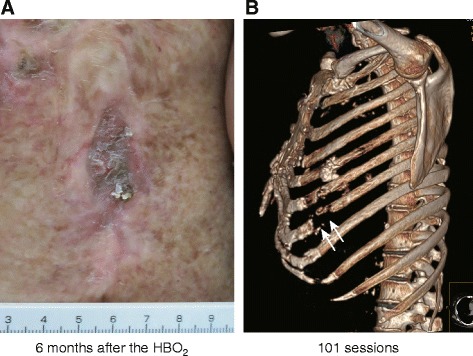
Gross appearance of the affected area and three-dimensional computed tomographic image of the left chest wall 6 months after starting hyperbaric oxygen therapy (HBO_2_). a The fistula completely disappeared. b Three-dimensional computed tomographic image showing a rib defect (*arrows*)
